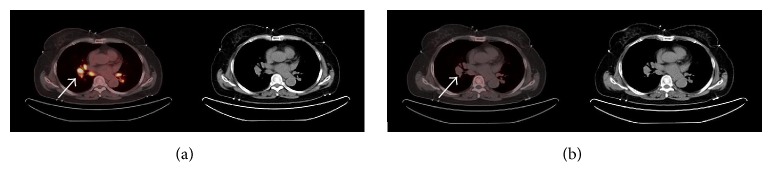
^18^F-FDG (a) and ^18^F-Alfatide II (b) PET/CT images of a sarcoidosis patient. All lesions showed intense ^18^F-FDG uptake and no ^18^F-Alfatide II uptake. The white arrows indicate sarcoidosis lesions in mediastinum and hilus pulmonis.
